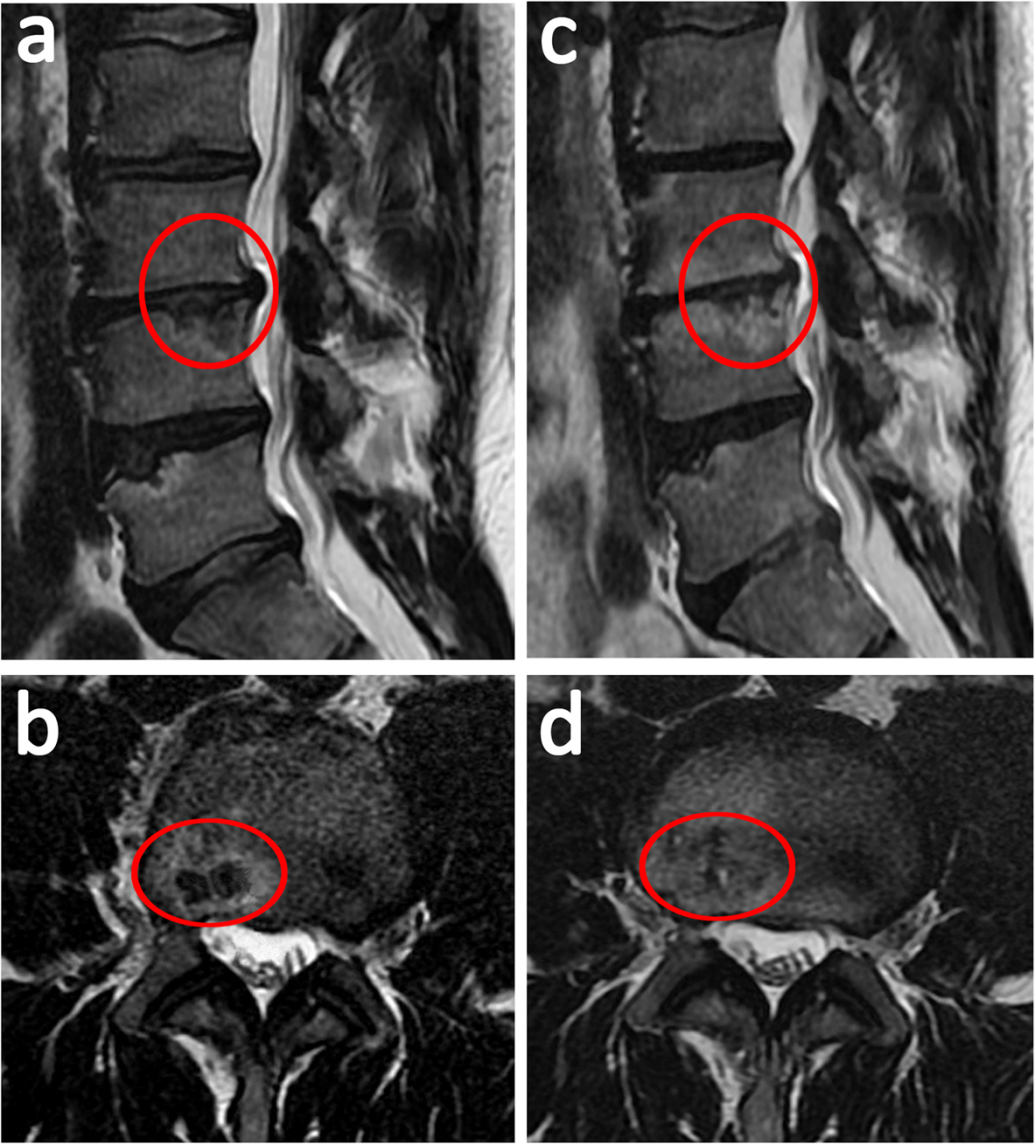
MRI scan before and after platelet-rich plasma treatment. Initial MRI a sagittal and b axial T2-weighted images (T2WI) (in yellow letters). Six months later, a substantial reduction of Schmörl’s node diameter (SND) was shown on c sagittal and d axial T2WI at the lumbar spinal level L3/L4. Red circles delineate SN size
